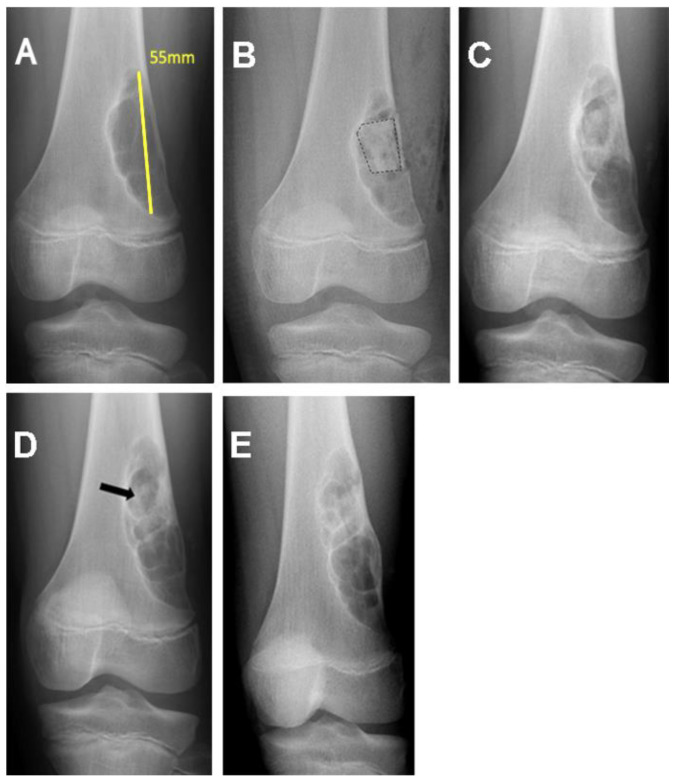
(A) Preoperative radiograph of a nonossifying fibroma of the distal femur. (B) Immediate postoperative radiograph after curettage showing poor cavity filling with bone graft. Dashed lines delimit the grafted area. (C) Postoperative 6-month follow-up radiograph showing persistent radiolucent areas > 50% of the bone diameter (Neer III) and (D) 12-month follow-up radiograph with remnants of the graft in the proximal region of the cavity (arrow). (E) Twenty-four-month follow-up radiograph showing persistent cystic areas and complete resorption of the bone graft.
